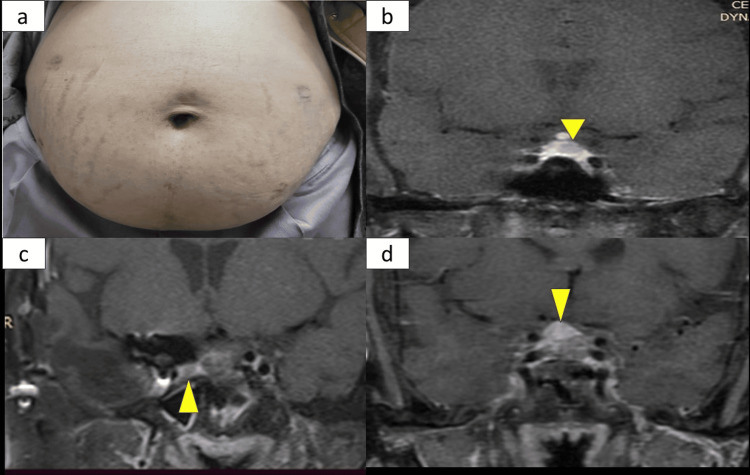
(a) Case 1: Thin purplish striae over the patient's abdomen. (b) Case 1: post-contrast T1 weighted MRI image suggestive of pituitary microadenoma (yellow arrow). (c) Case 2 post-contrast T1 weighted CEMR of pituitary and hypothalamic area suggestive of residual pituitary microadenoma (yellow arrow) with distorted sellar anatomy due to previous surgery. (d) Case 3: Post-contrast T1-weighted CEMR pituitary and hypothalamic area suggestive of pituitary macroadenoma (yellow arrow) CEMR: Contrast-enhanced magnetic resonance imaging
